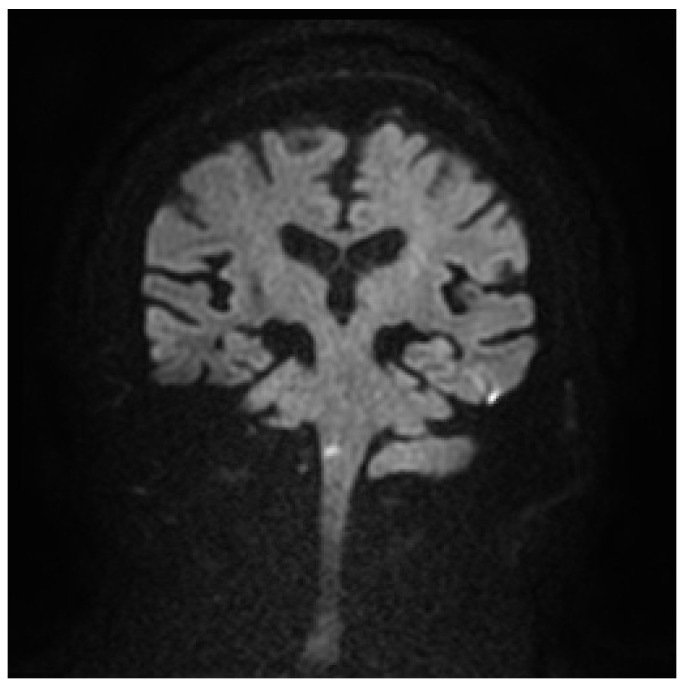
Diffusion-weighted magnetic resonance imaging showed evidence of acute brainstem infarction in the right lateral medulla.
